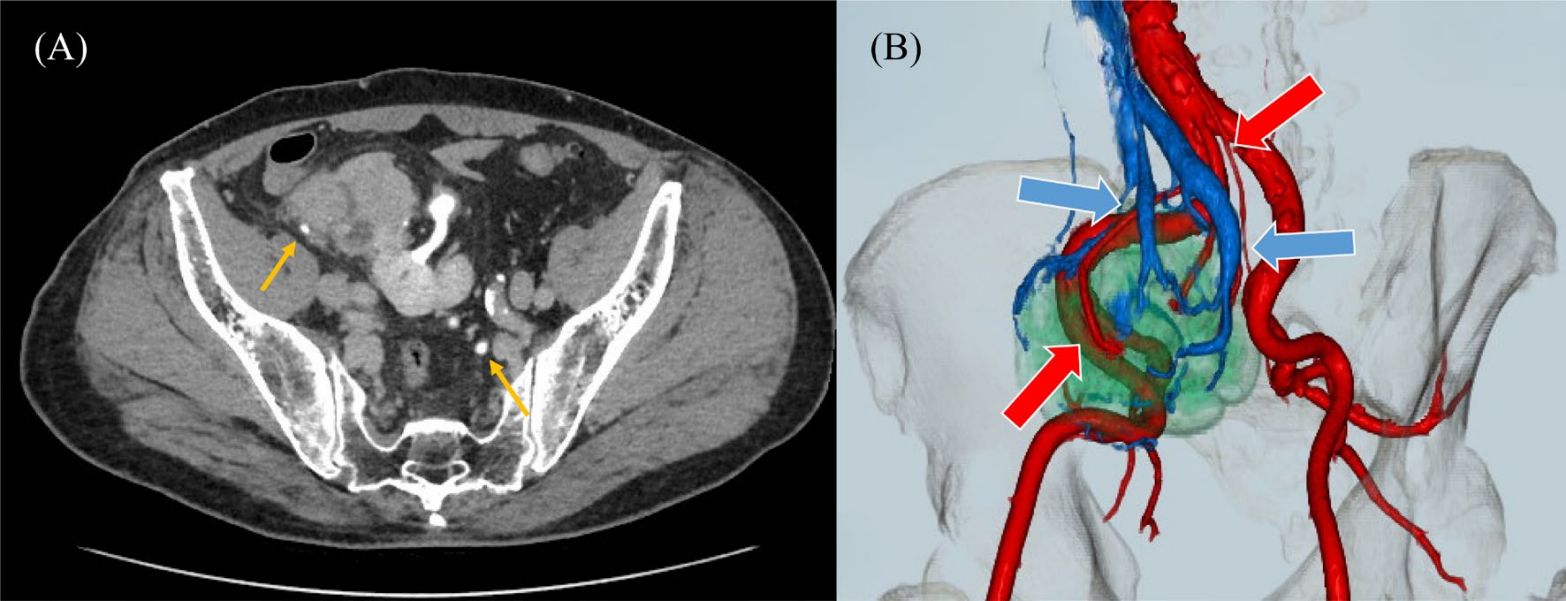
(A) The right ureter passes posterior to the left pelvic kidney, while the left ureter descends anteriorly (yellow arrows). (B) Three‐dimensional CT angiography showing anomalous renal vasculature. The left renal artery originates from the abdominal aorta and right common iliac artery (red arrows); multiple renal veins drain into the IVC (blue arrows). CT, computed tomography; IVC, inferior vena cava.
